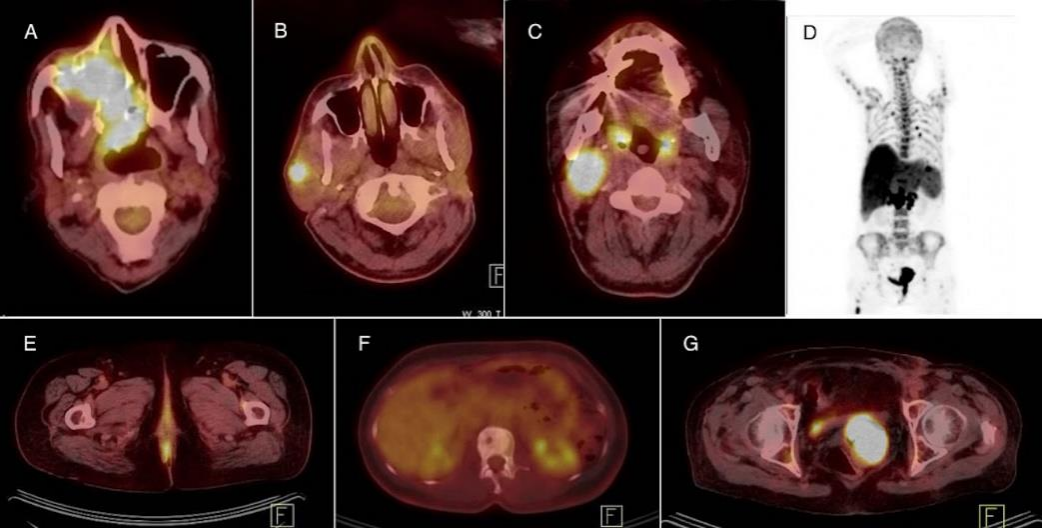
(A) FDG PET/CT of an 87-year-old male with noncutaneous melanoma (NCM) in the right nasal cavity with extension to adjacent sinuses (case 9). (B) FDG PET/CT of a 54-year-old male with NCM, presenting after resection of left parotid mass. (Additional metastatic lesion is noted in the right parotid, not recognized on patient’s CT scan, case 21). (C) A 40-year-old male with NCM of right tonsil. (FDG PET/CT reveals residual disease at the site of right tonsillectomy as well as metastatic lymph nodes in right cervical zone II, case15). (D) MIP image of a 74-year-old female with vulvar NCM revealing extensive metastases, case 20. (E) FDG PET/CT of a 66-year-old female with NCM in the anal canal (case 18). (F) FDG PET/CT image of a 32-year-old female with NCM of unknown primary currently revealing metabolically inactive sclerotic bone lesions in the site of previously lytic bone metastases, indicative of complete response to treatment (case 22). (G) FDG PET/CT image of the patient in MIP image (D), revealing local recurrence (case 20)
